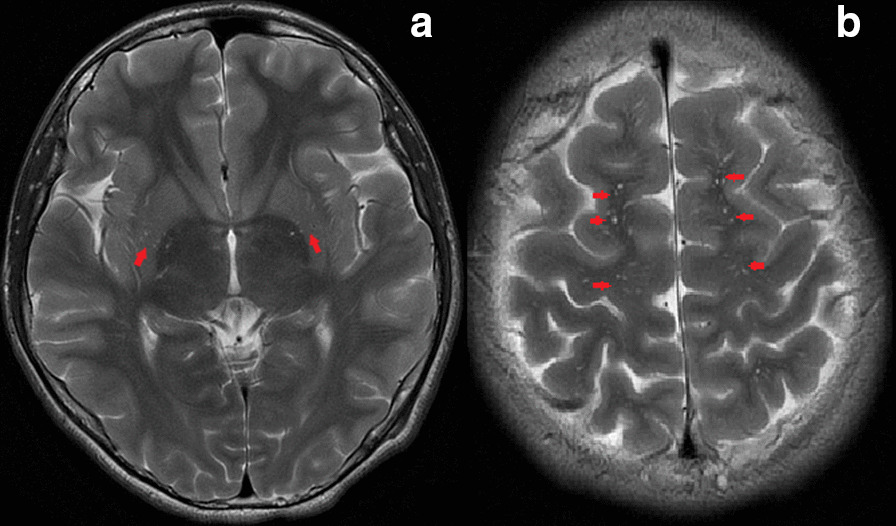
Magnetic resonance imaging (MRI) data obtained from the brother with CYP7B1 deficiency, T2-weighted images show massively enlarged perivascular spaces (showed by red arrows) in the bilateral basal ganglia (a) and white matter of frontal parietal (b)
